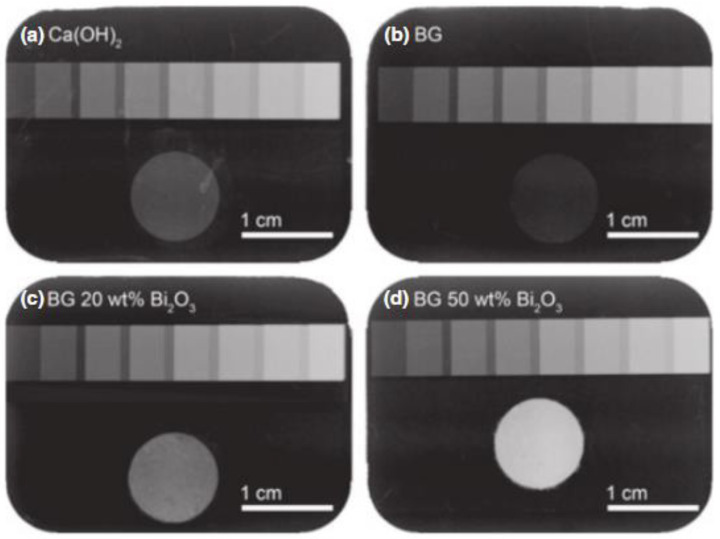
Radiography image of melt-derived BGs and aluminum-scale reference material: (a) calcium hydroxide, (b) 45S5 Bioglass^®^, (c) 45S5 Bioglass^®^ with 20 wt.% of Bi_2_O_3_, and (d) BG with 50 wt.% of Bi_2_O_3_ [145].
